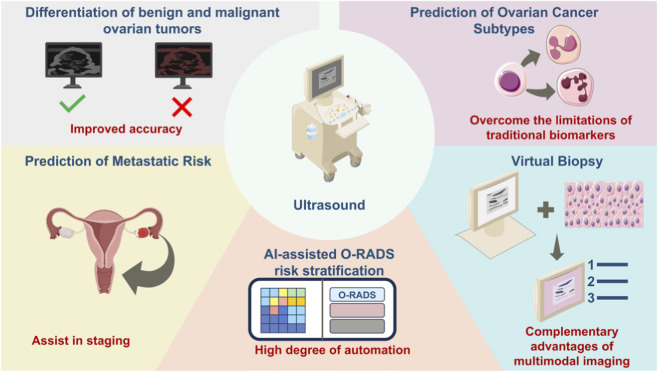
Applications of ultrasound-based radiomics in ovarian cancer management. The major clinical applications of ultrasound-based radiomics in ovarian cancer, including tumor differentiation, AI-assisted O-RADS risk stratification, subtype prediction, metastatic risk assessment, and virtual biopsy. By integrating imaging features with artificial intelligence and clinical data, these approaches enhance diagnostic accuracy, automate risk classification, and support individualized treatment planning. Reproduced with permission from (cf. Huang et al., 2025), copyright 2025 ©The Author(s).
